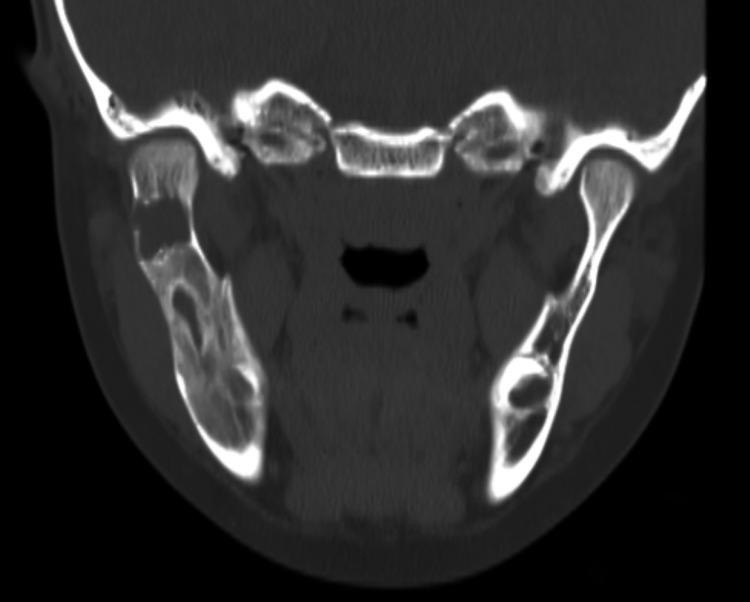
Post-marsupialization CT of the mandible
